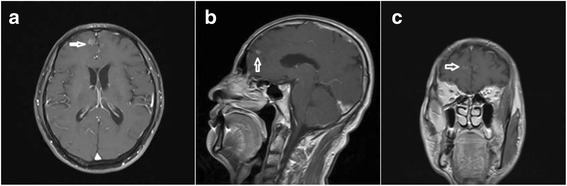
Arrow indicates very clear enhanced shadow in a and b and c MRI images
